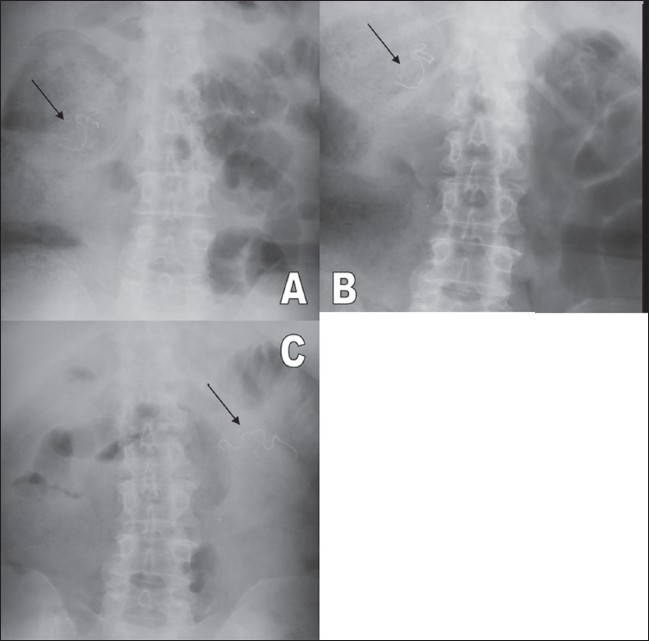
Plain abdominal radiographs show migration of the radiopaque marker (black arrows) from the right upper quadrant (A), moving upwards towards the center (B) and then to the left upper quadrant (C)
